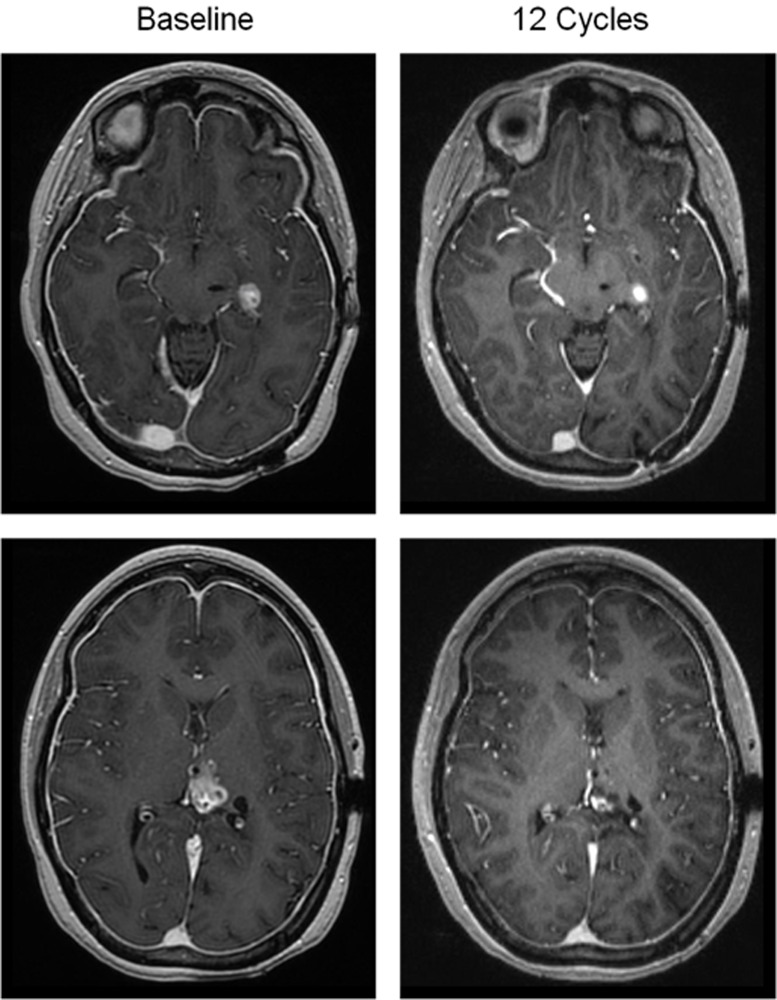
Objective response in a patient with recurrent glioblastoma T1- post contrast brain MRI reveals two enhancing lesions at baseline (left top and bottom panels), which are decreased in size following 12 cycles of therapy (36 weeks; right top and bottom panels).
